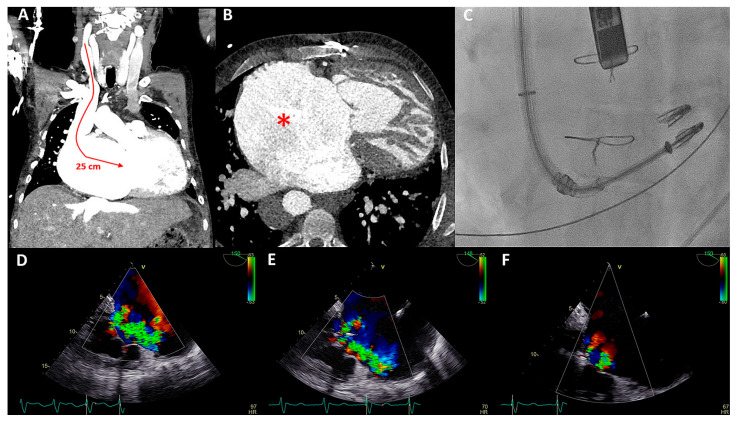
(A) Distance between the right internal jugular vein and tricuspid valve (TV) measured on a computed tomography (CT) scan in a coronal plane (arrow). (B) Severely dilated mono-atrium (asterisk) on CT scan in a sagittal plane. (C) Implantation of the second XTW clip (note the first XTW clip already released) under transesophageal echocardiography (TEE) guidance. (D) Preprocedural TEE imaging of the torrential (IV+) TR. (E) Periprocedural TEE showing TR after placing the first XTW clip. (F) TEE showing the significant reduction in TR after placing the second XTW clip to grade I–II.
